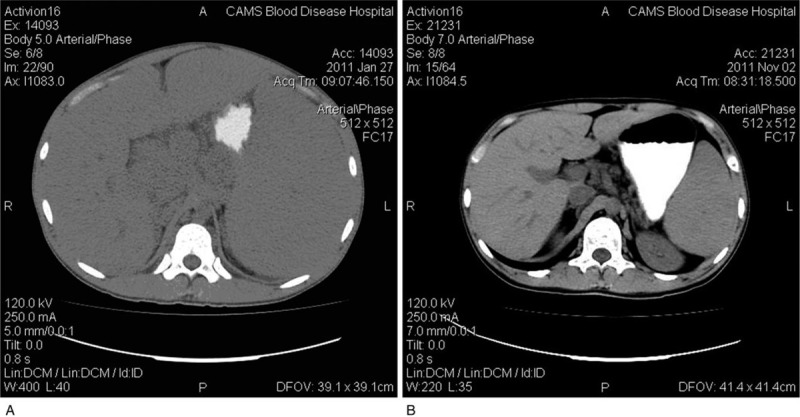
A. Abdomen CT image before treatments in patient 2: enlargements of intraperitoneal lymph nodes, liver and spleen. 1B. Abdomen CT image 3 mo after ASCT in patient 2: intraperitoneal lymph nodes, liver and spleen returned to the normal size. ASCT = autologous stem cell transplantation, CT = computed tomography.
